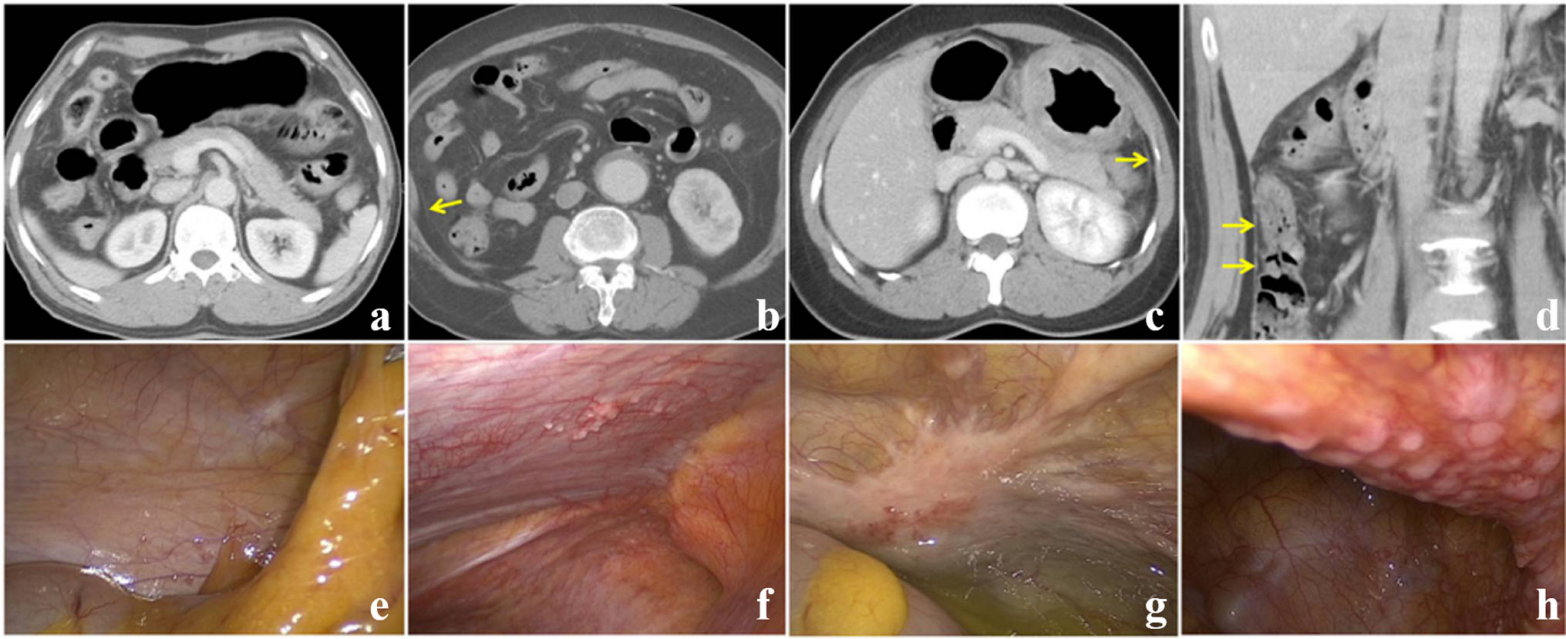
Occult peritoneal metastasis (OPM) score of the peritoneum attached to the organs or tissues. a OPM grade 0 (no thickening of the peritoneum observed). b OPM grade 1 (slight thickening with a smooth contour). c OPM grade 2 (obvious thickening with enhancement). d OPM grade 3 (obvious thickening with enhancement and tiny nodules). e–h Intraoperative screen shots of staging laparoscopy corresponding to the aforementioned computed tomography (CT) findings. The degree of metastasis is displayed from light to severe
